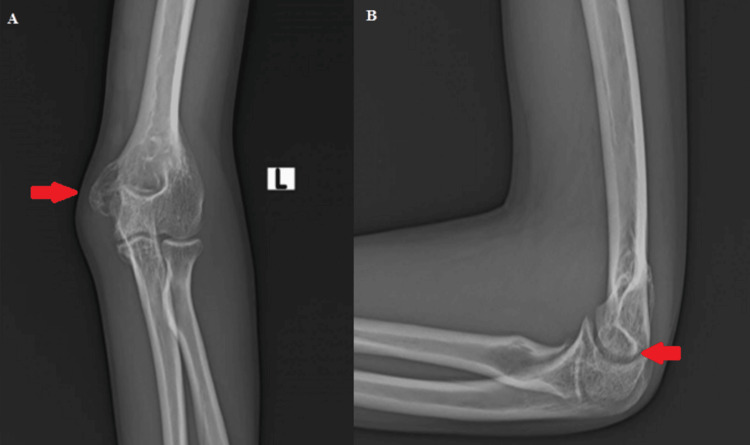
Postoperative X-rays of the left elbow joint were taken on the second day to assess the extent of debridement A: anteroposterior view; B: lateral view
